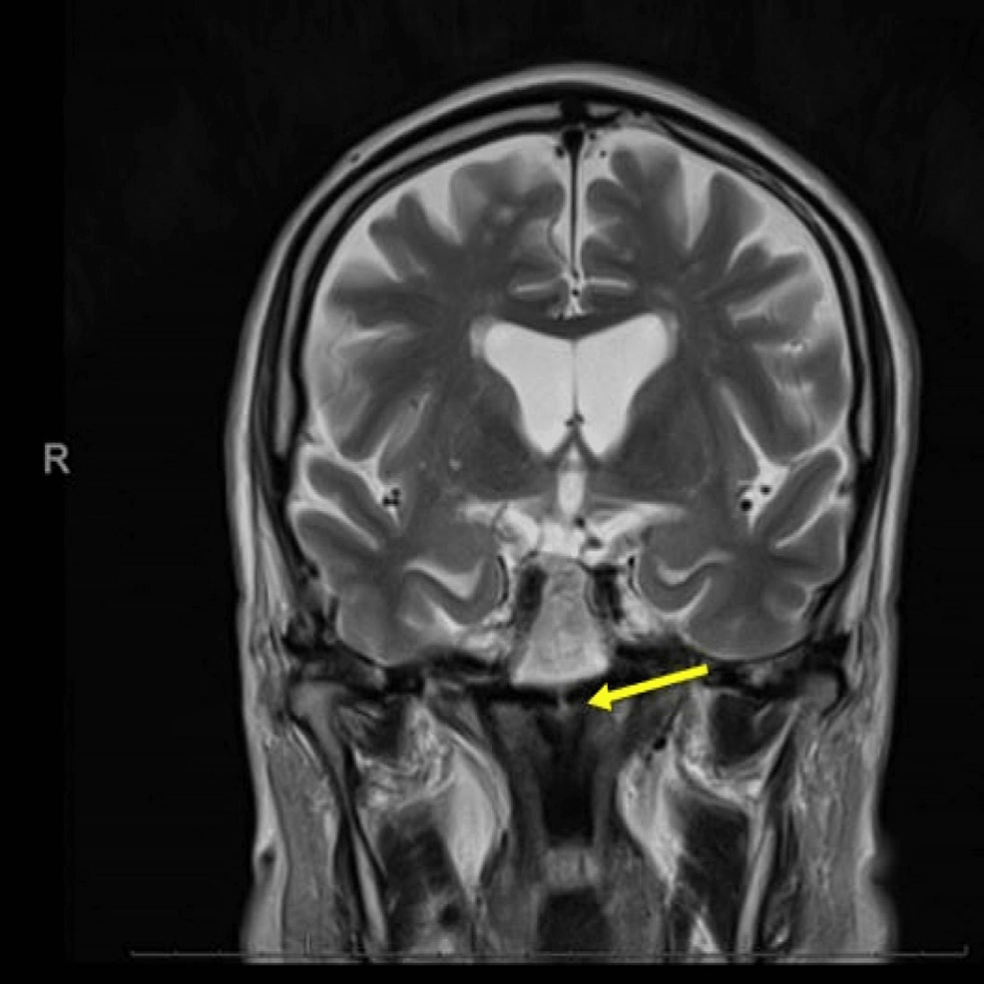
Magnetic resonance imaging of the brain (coronal view): re-demonstration of restricted diffusion in the left paramedian tegmentum (yellow arrow).
